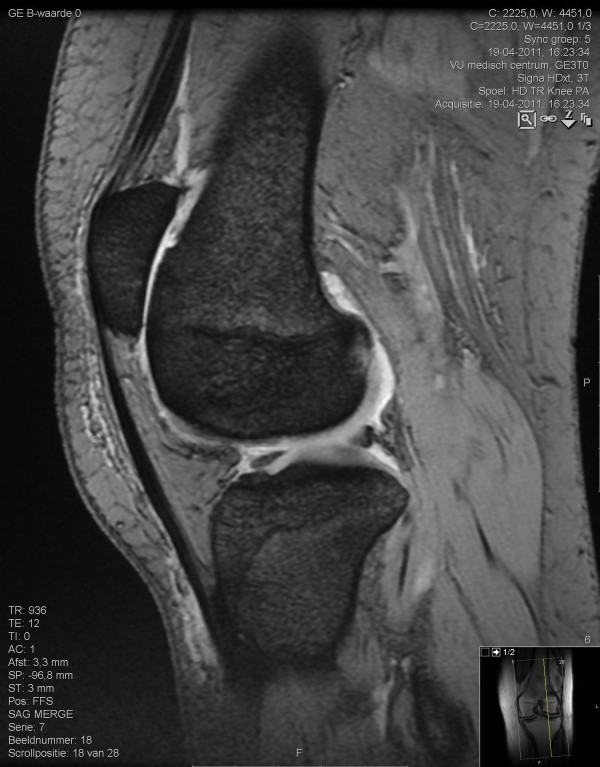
Reduced cartilage integrity in the patellafemoral compartment. Sagittal combined multi-echo gradient echo 3.0 Tesla magnetic resonance imaging scan.
